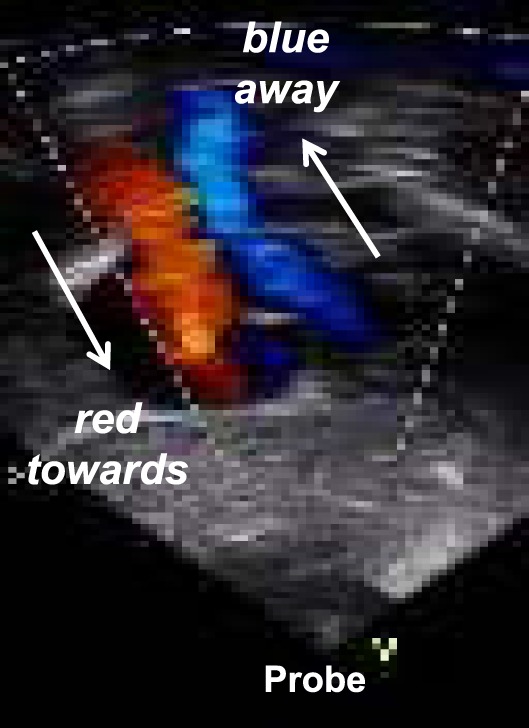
Color Doppler flow mapping with Blue directed away from the probe and red directed toward the probe: mnemotechnic = BART.
